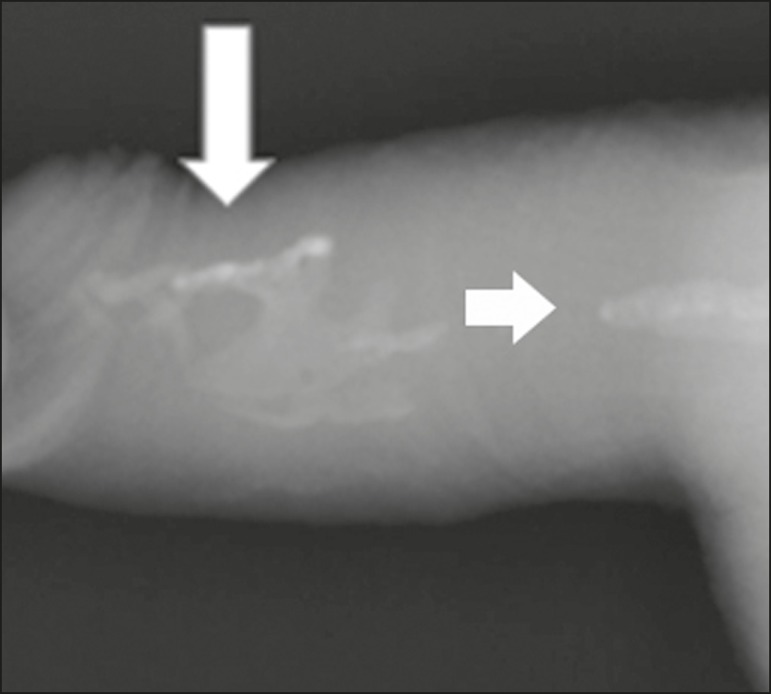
Conventional X-ray, penetrating the soft parts of the penis, showing
